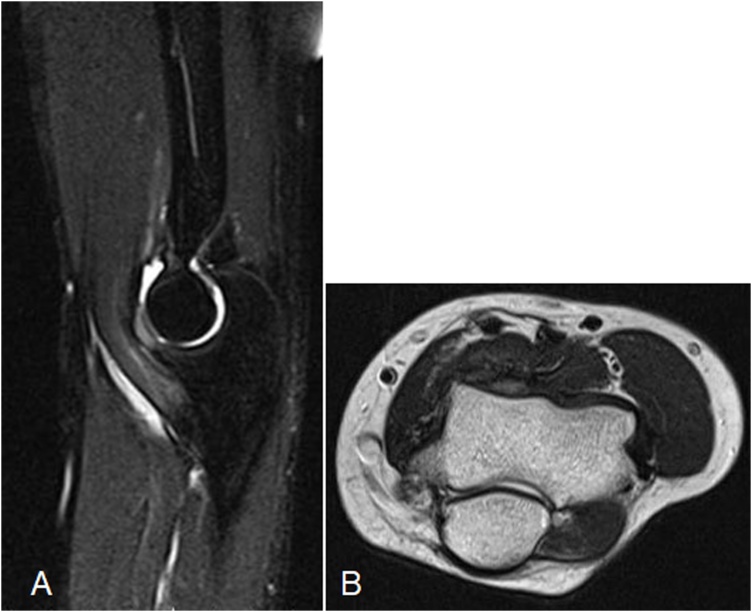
Final axial T1-weighted (A) and sagittal (B) magnetic resonance images.
